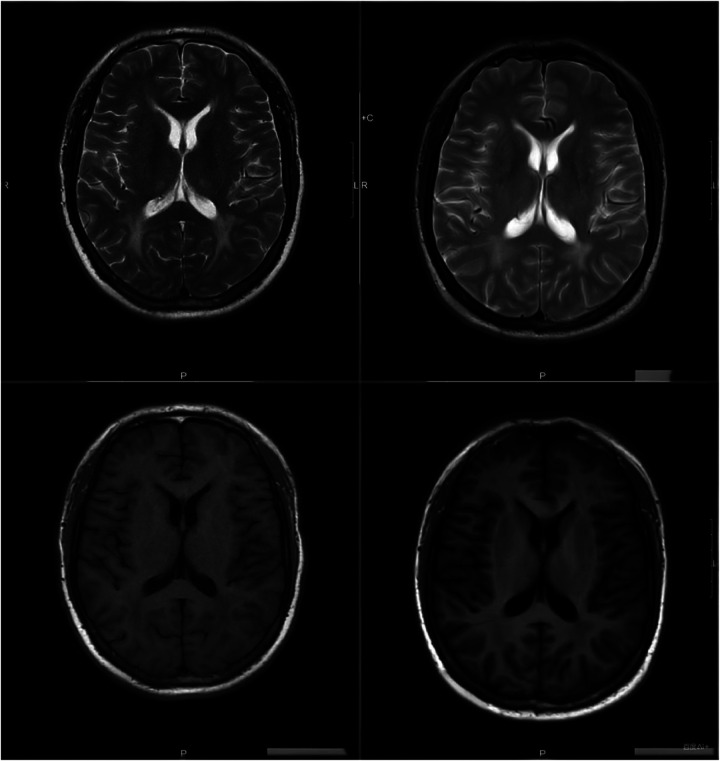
Comparison of head MRI findings before and after inpatient treatment: 1. The bilateral lateral ventricle, half oval center, corona radial, and bilateral frontoparietal lobe. 2. Puntate abnormal signals in the bridge and right cerebellar hemisphere, same with the former, considering the possibility of old bleeding lesions; 3. Small patchy abnormal signal lesions in bilateral basal ganglia and left lateral ventricle, same with the former, considering old lacunar infarction lesions.
